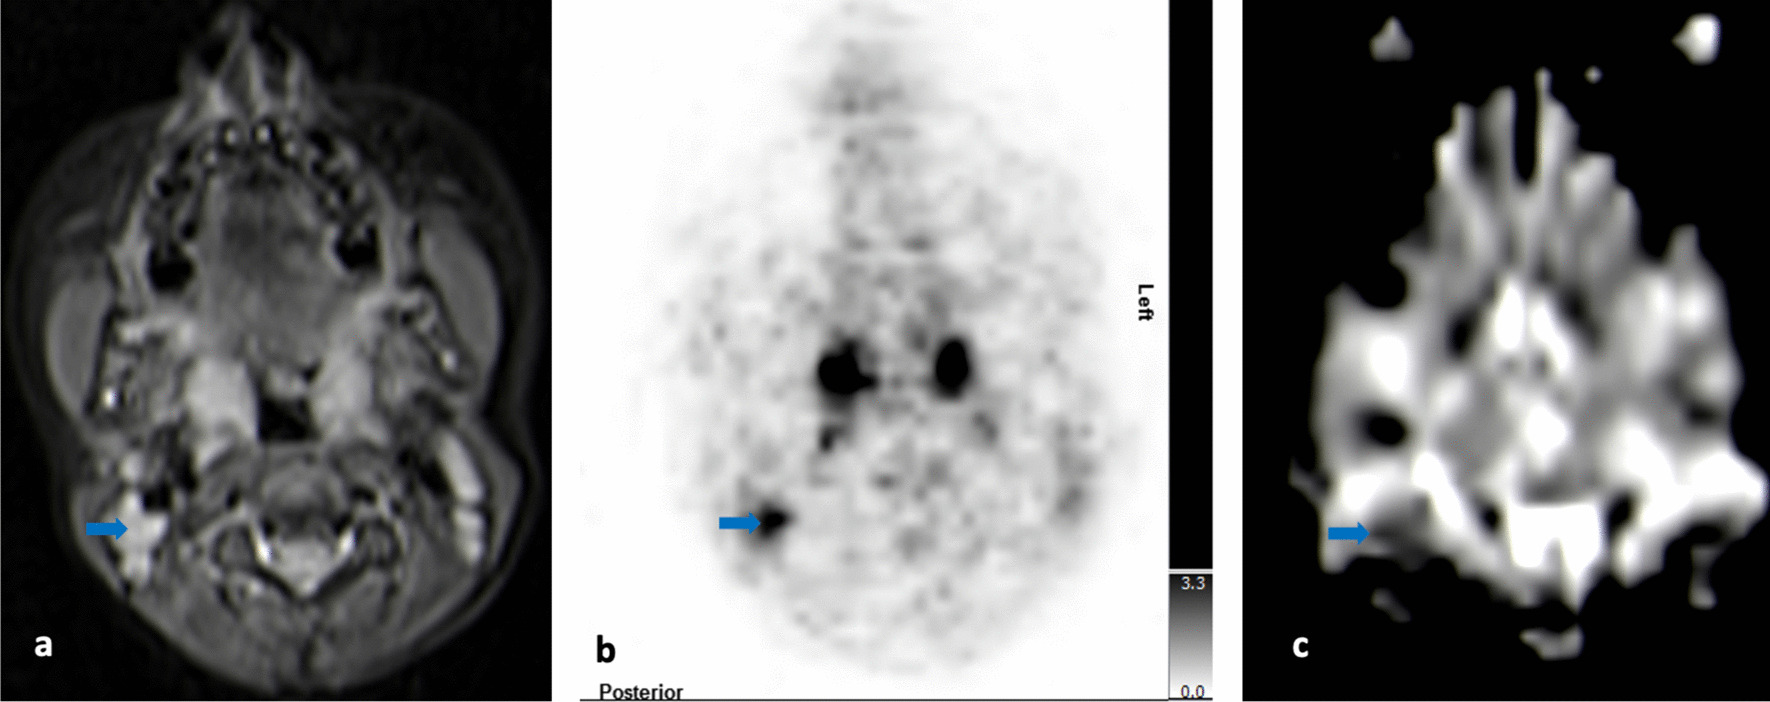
a–c Upper neck (level II) on T2-TIRM transversal (a), [^18^F]-FDG-PET (b), and DWI at b-value of 800 (c): The blue arrows point to morphologically inconspicuous lymph nodes on the right side (a) with increased glucose metabolism (SUV_max_ right: 3.96 versus SUV_max_ left: 1.84) (b) and restricted diffusion (ADC right on average: 643 × 10^–6^ mm^2^/s versus ADC left on average: 1480 × 10^–6^ mm^2^/s) (c)
